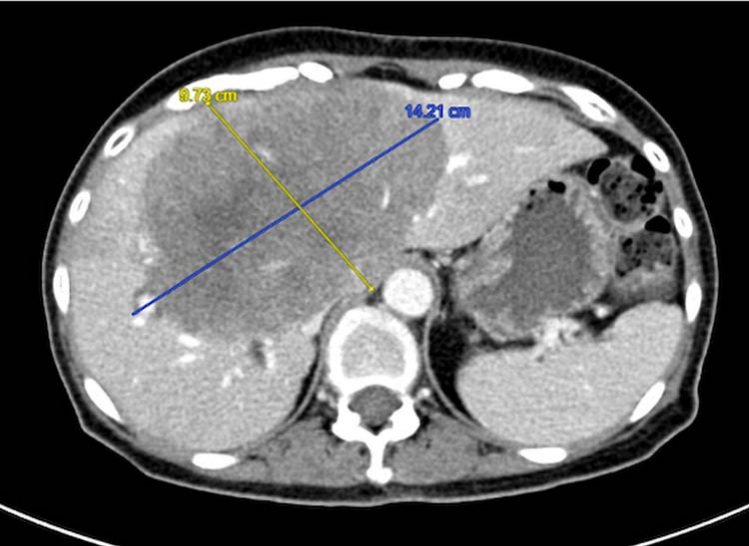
Axial CT scan demonstrating liver metastasis post 4 cycles of chemotherapy.
